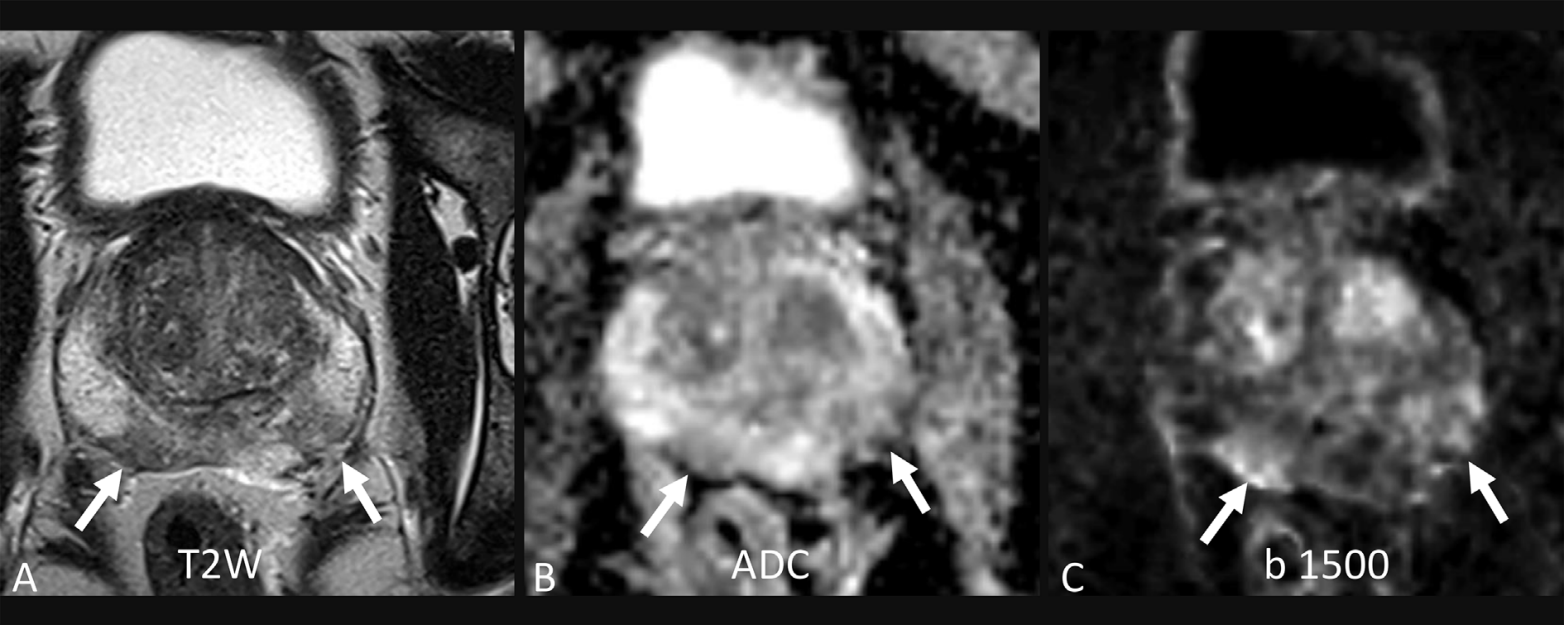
Siemens Healthcare AG (Erlangen, Baviaria, Germany) VIDA OPT prostate MRI (3 T) with (A) bilateral T2W hypointensities and (B and C) mildly restricted diffusion with ADC values >1200 × 10^–6^ mm^2^/s. The primary reader assigned bilateral PI-RADS 4 lesions; both central review readers assigned PI-RADS 2. Two rounds of bilateral targeted biopsies (six cognitive biopsies in the first round and eight TRUS/MRI fusion biopsies in the second round) showed benign prostate tissue only. A repeat MRI scan 2 yr later in the OPT program was assessed to be PI-RADS 2. ADC = apparent diffusion coefficient; MRI = magnetic resonance imaging; OPT = prostate cancer testing; PI-RADS = Prostate Imaging Reporting and Data System; TRUS = transrectal ultrasound; T2W = T2 weighted.
